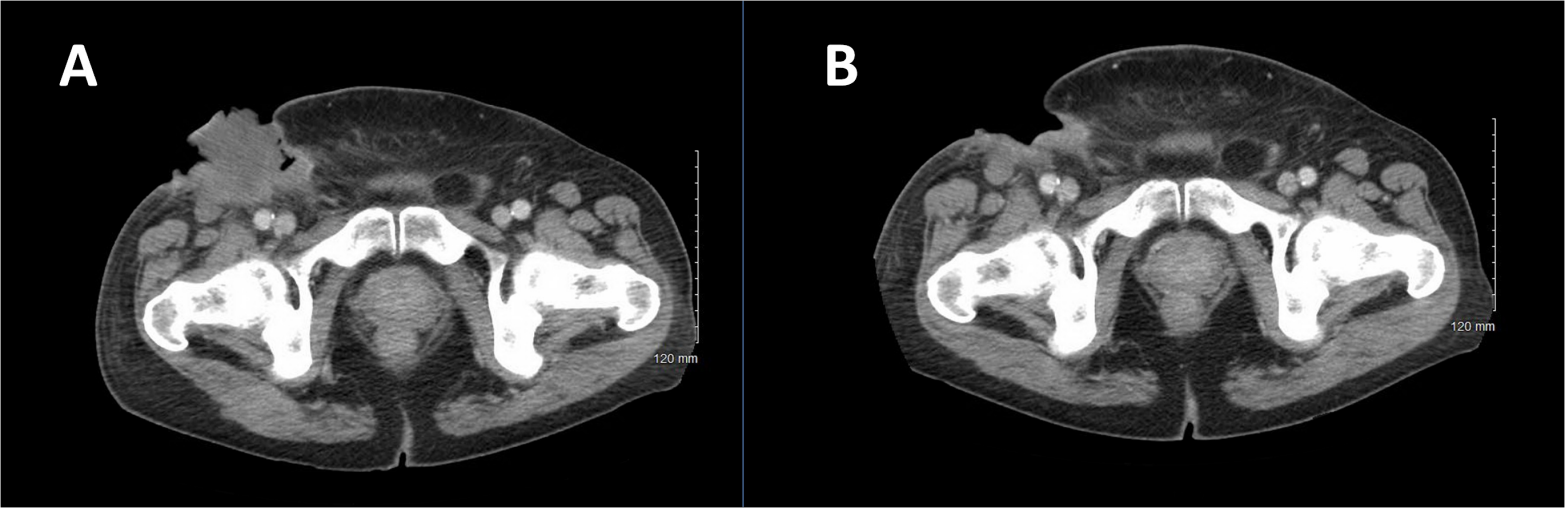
CT Scan of the Abdomen and Pelvis Before and After Six Weeks of BRAF-targeted Therapy A: CT scan of the abdomen and pelvis prior to initiation of dabrafenib/trametinib therapy. B: Regression of primary mass after six weeks of BRAF targeted therapy. Surgical excision of the remnant mass confirmed no evidence of residual sarcoma.
